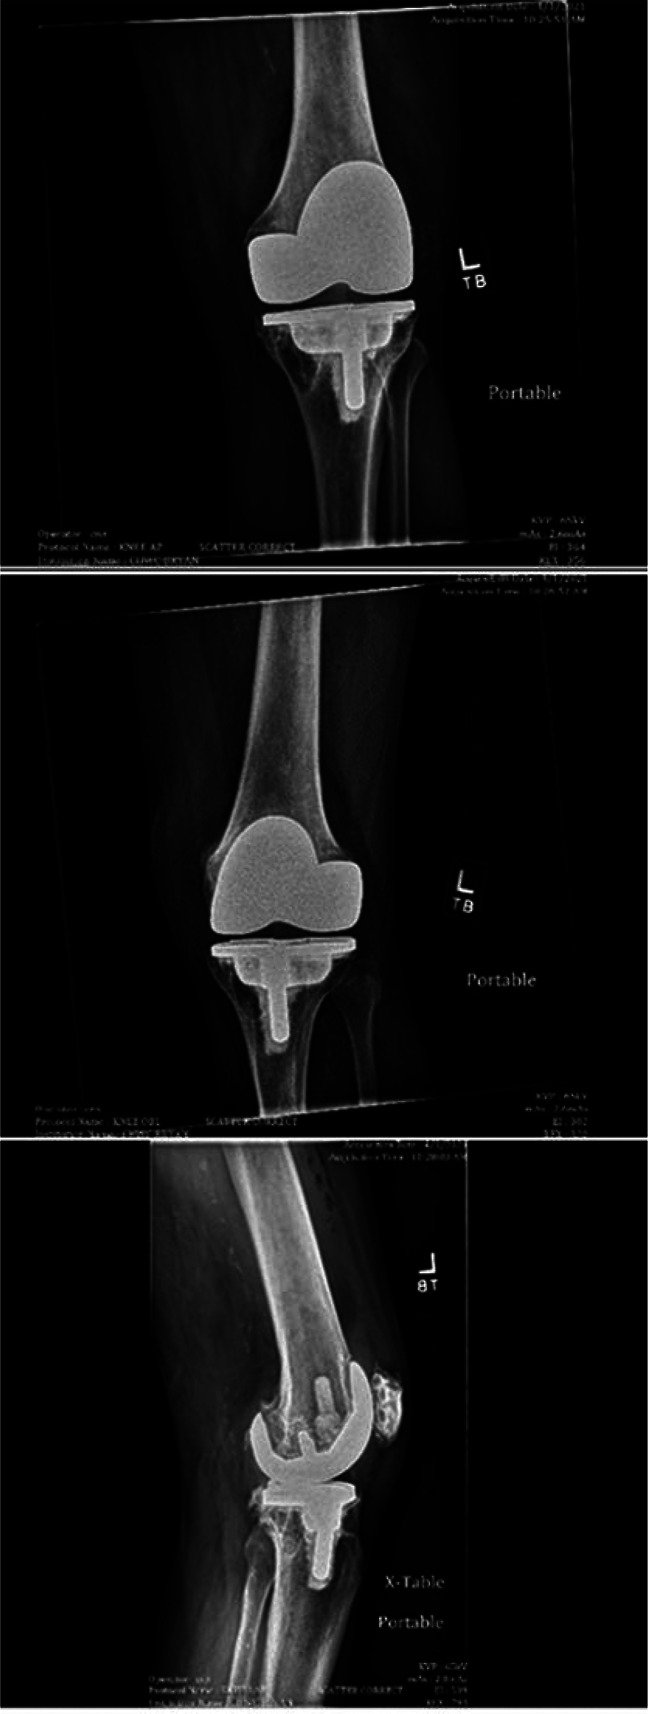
A, Anteroposterior (AP), (B) oblique, and (C) lateral radiographic views of left total knee arthroplasty at initial presentation showing soft-tissue swelling.
